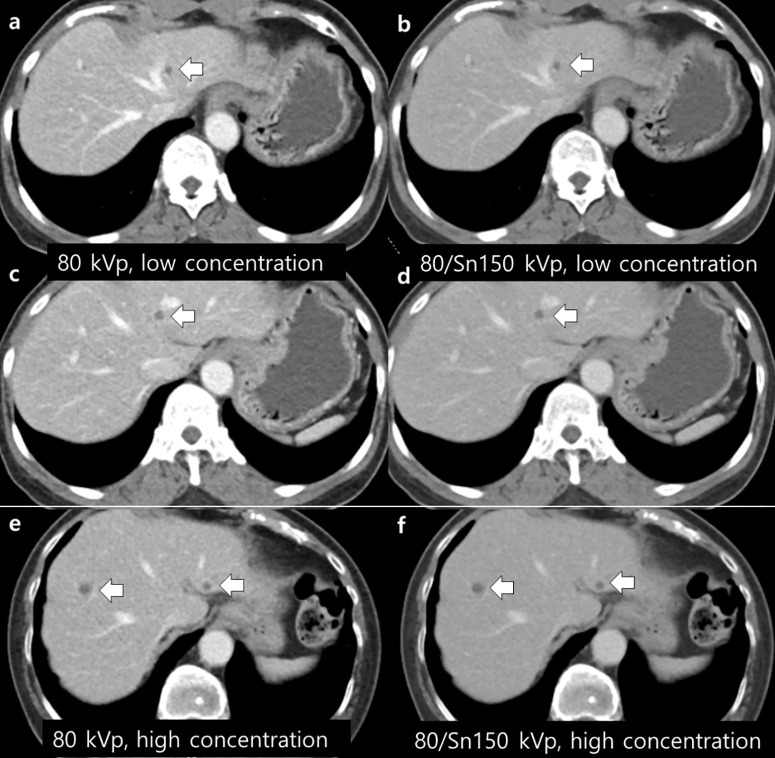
Abdominopelvic computed tomography (CT) images of two patients with breast cancers. CT images of two patients with breast cancer (64-year-old, female; weight, 57.0 kg; body mass index, 21.7 kg/m^2^; a-b) vs (80-year-old, female; weight, 52 kg; body mass index, 23.1 kg/m^2^; c-d) with matching cases. (a-d) CT images show two hepatic cysts (arrows, 5 and 6 mm in size, respectively) using a low concentration of the contrast agent, and (e-f) two hepatic cysts (arrows, 5 and 7 mm in size, respectively) using a high concentration of the contrast agent. The overall image quality was scored 3 for the 80 kVp CT image (a and c), 4 for the 80/Sn 150 kVp image (b and d), 3 for the 80 kVp CT image (e), and 3 for the 80/Sn 150 kVp image (f). The conspicuity and margin sharpness of the hepatic lesions were similar, with a score of 4 for both parameters in all images.
